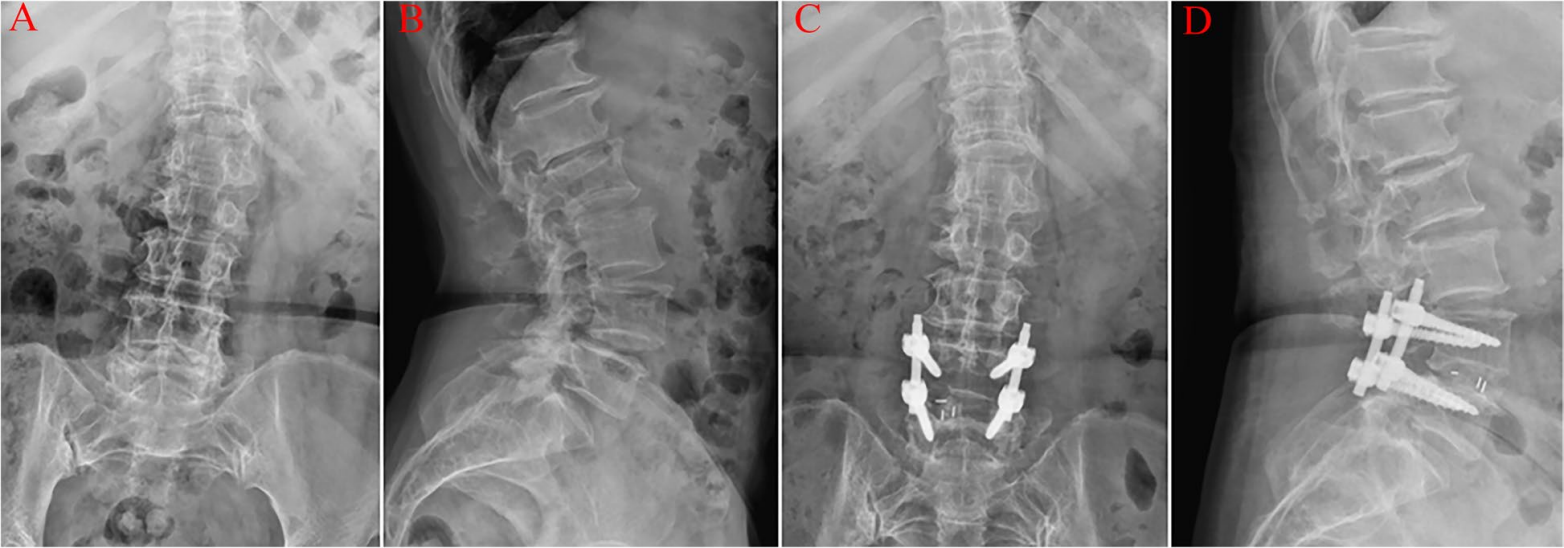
A 66-year-old female patient. A and C are the frontal X-rays of the lumbar spine before and after the operation, whereas B and D are the lateral X-rays of the lumbar spine before and after the operation
